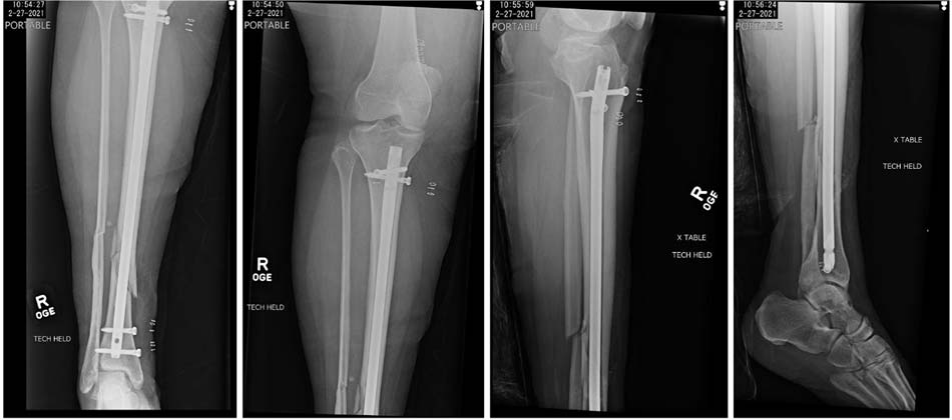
Postoperative radiographs demonstrating reduction of distal tibia/fibula fracture.
